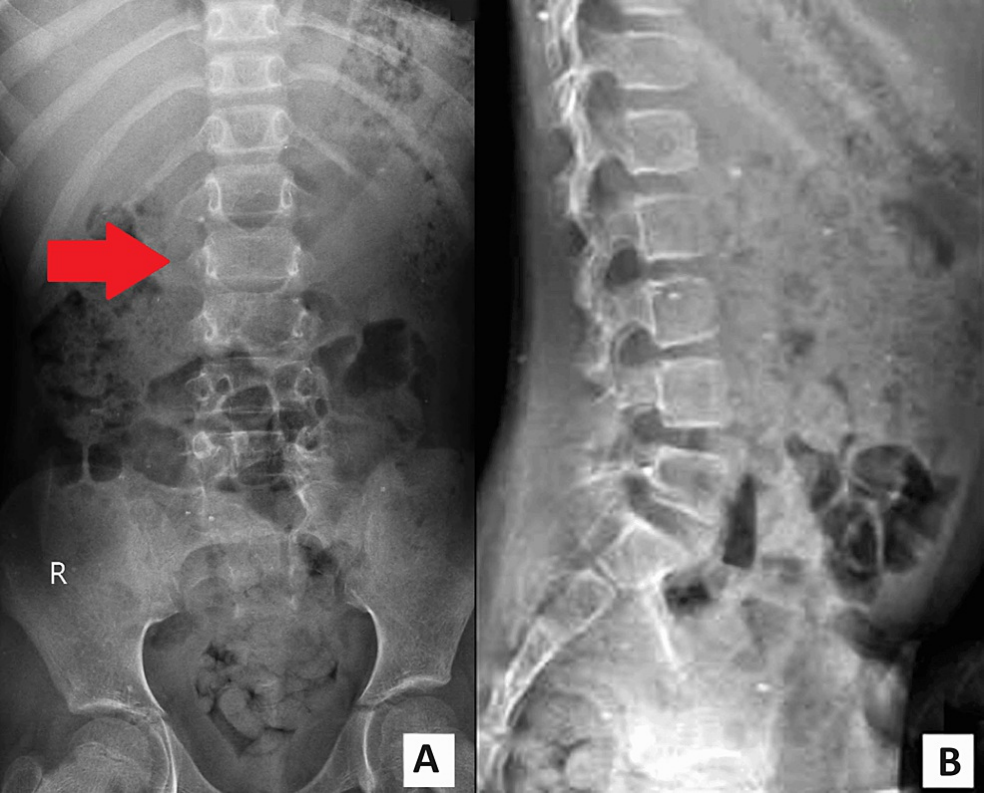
A and B show the X-Ray lumbar spine (AP and lateral) showing the bifid spinous process at L3, L4, and L5 levels AP: Anteroposterior
